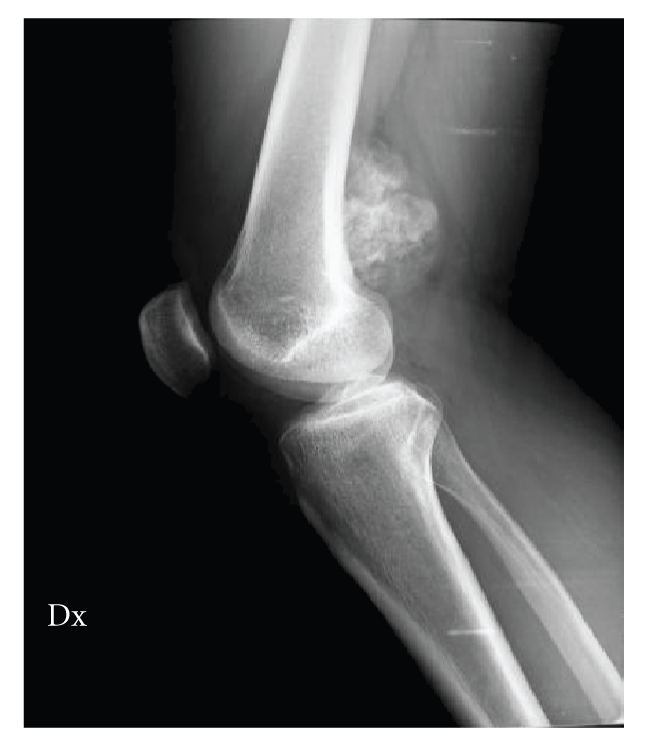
Preoperative radiography showed a relatively dense and well-demarcated ossified mass at a
